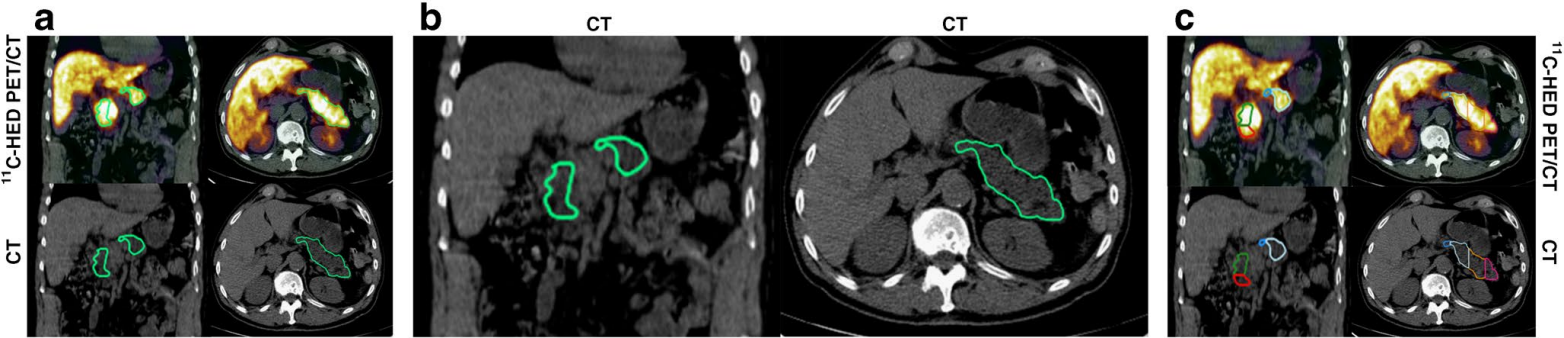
(a) ^11^C-HED-PET/CT and CT scans showing delineation of the whole pancreas, applying 41% of the maximum activity cut-off VOI (outlined in green). (b) Division of the pancreas was performed manually in the coronal and transversal planes on CT scans to obtain six pancreatic regions. (c) Uptake parameters were obtained from the pancreatic regions on ^11^C-HED-PET/CT and CT. Red outlining indicates head caudal; green outlining indicates head cranial; blue outlining indicates body proximal; white outlining indicates body distal; orange outlining indicates tail proximal; and pink outlining indicates tail distal
